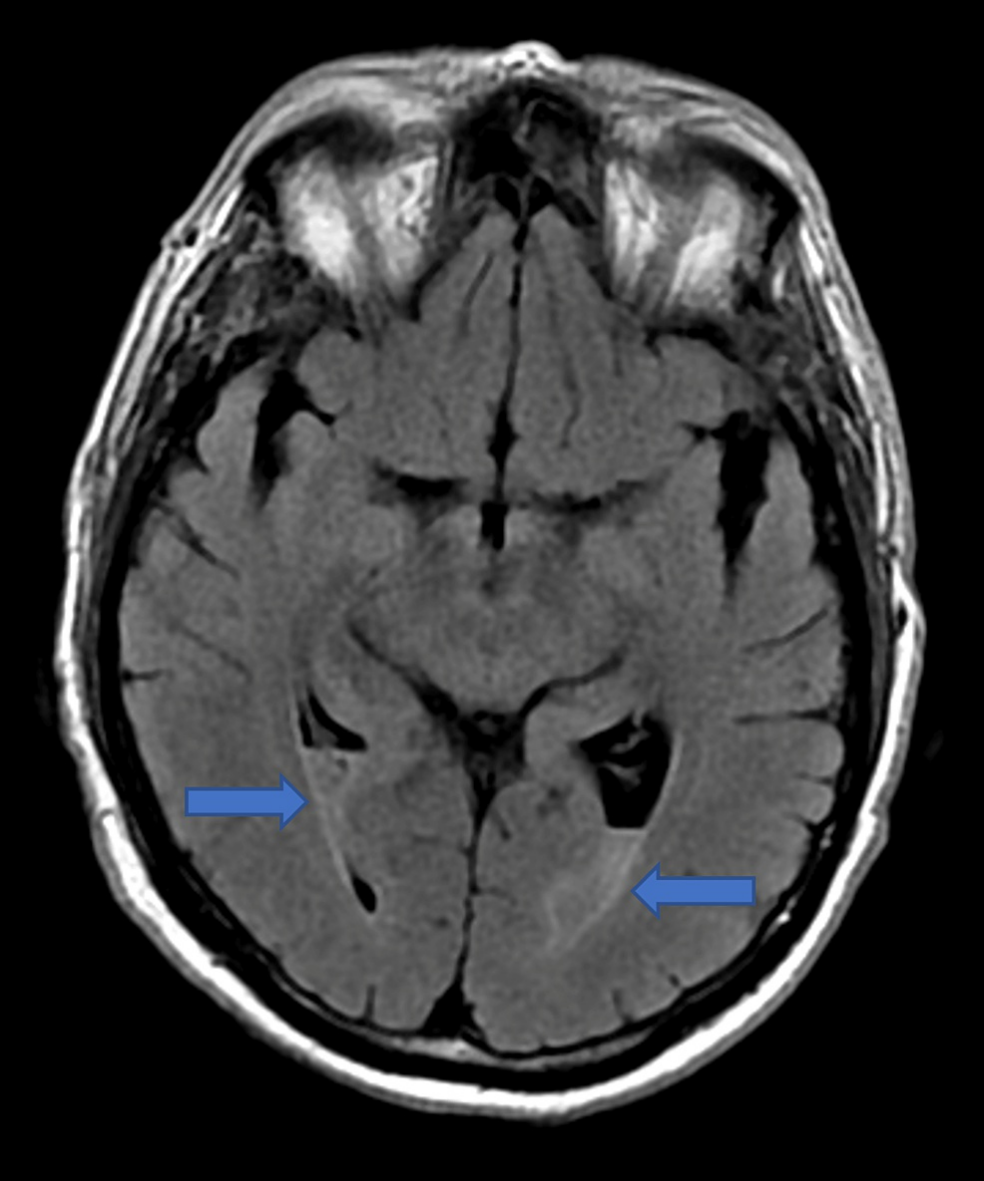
T2 fluid-attenuated inversion recovery (FLAIR) imaging demonstrating layering purulent debris within the bilateral occipital horns (blue arrows).
